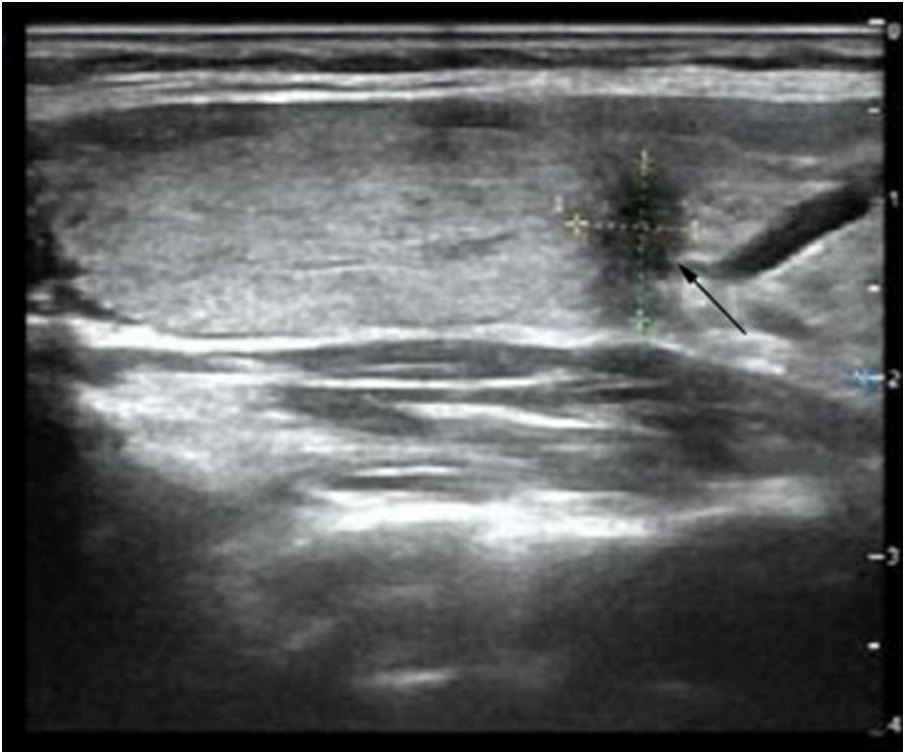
Ultrasound image expresses a thyroid nodule (0.70 cm × 0.70 cm × 0.90 cm) located at the inferior thyroid on the right side (shown by a black arrow).
